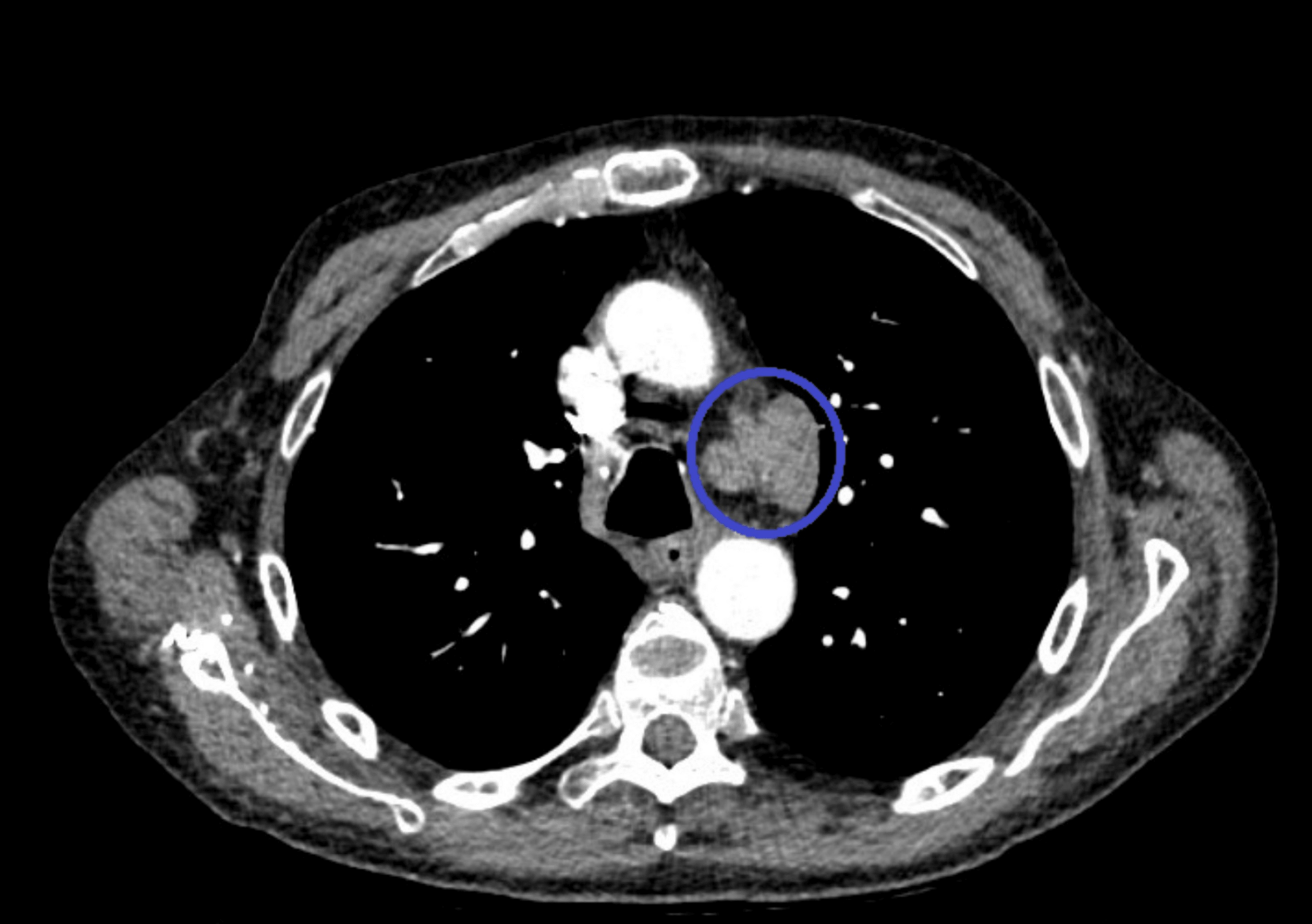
Repeated CT chest one year later showing disease progression CT: computed tomography
